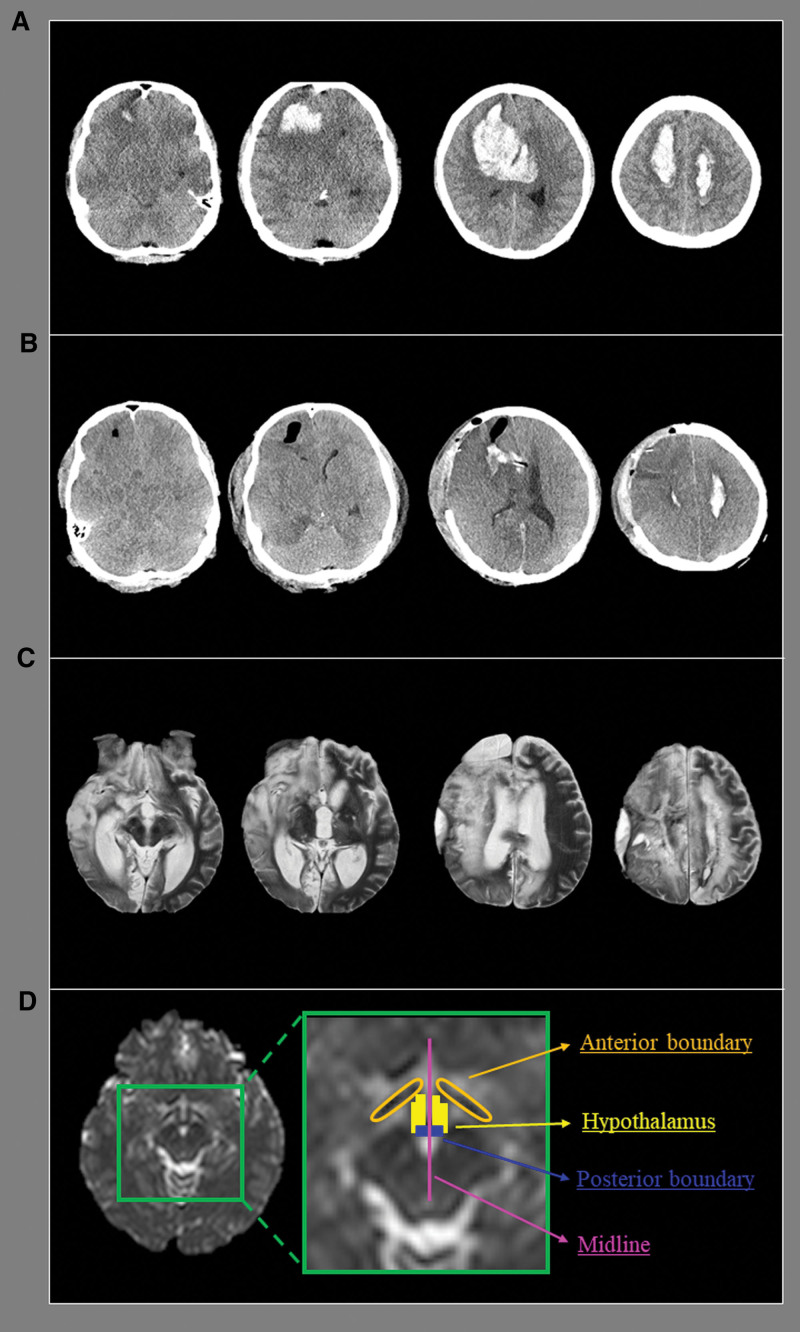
(A) Brain computed tomography images at the stroke onset show a spontaneous intraventricular hemorrhage and intracerebral hemorrhage. (B) Brain computed tomography images taken after the craniectomy and hematomal removal operation show aggravated brain edema. (C) Brain magnetic resonance images at 2 months after onset reveal leukomalatic lesions in both fronto-parieto-occipital cortices, right temporal lobe, and hypothalamus. (D) Regions of interest for the hypothalamus are localized using the optic tract (anterior boundary), the mammillary body (posterior boundary), and the midline (medial boundary) at the level of the upper midbrain in the patient.
